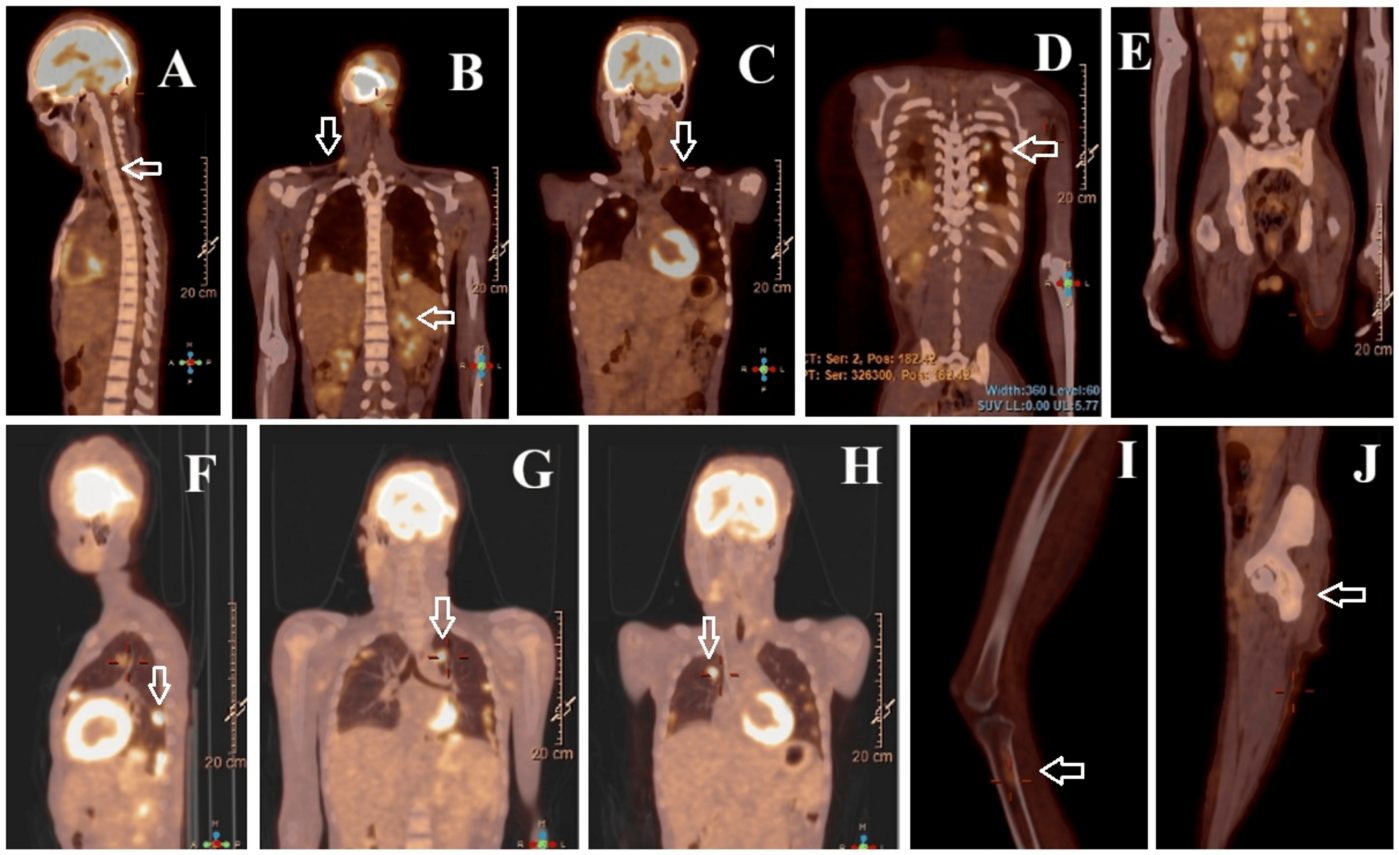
PET-CT images (coronal and sagittal views) demonstrating multiple foci of pathological FDG uptake (white arrows): (A) cervical vertebra metastasis, (B-C) cervical lymph nodes metastasis; (D-H) multiple lung metastases; (I-J) proximal right tibia metastasis FDG: Fluorodeoxyglucose; PET-CT: Positron emission tomography-computed tomography.
